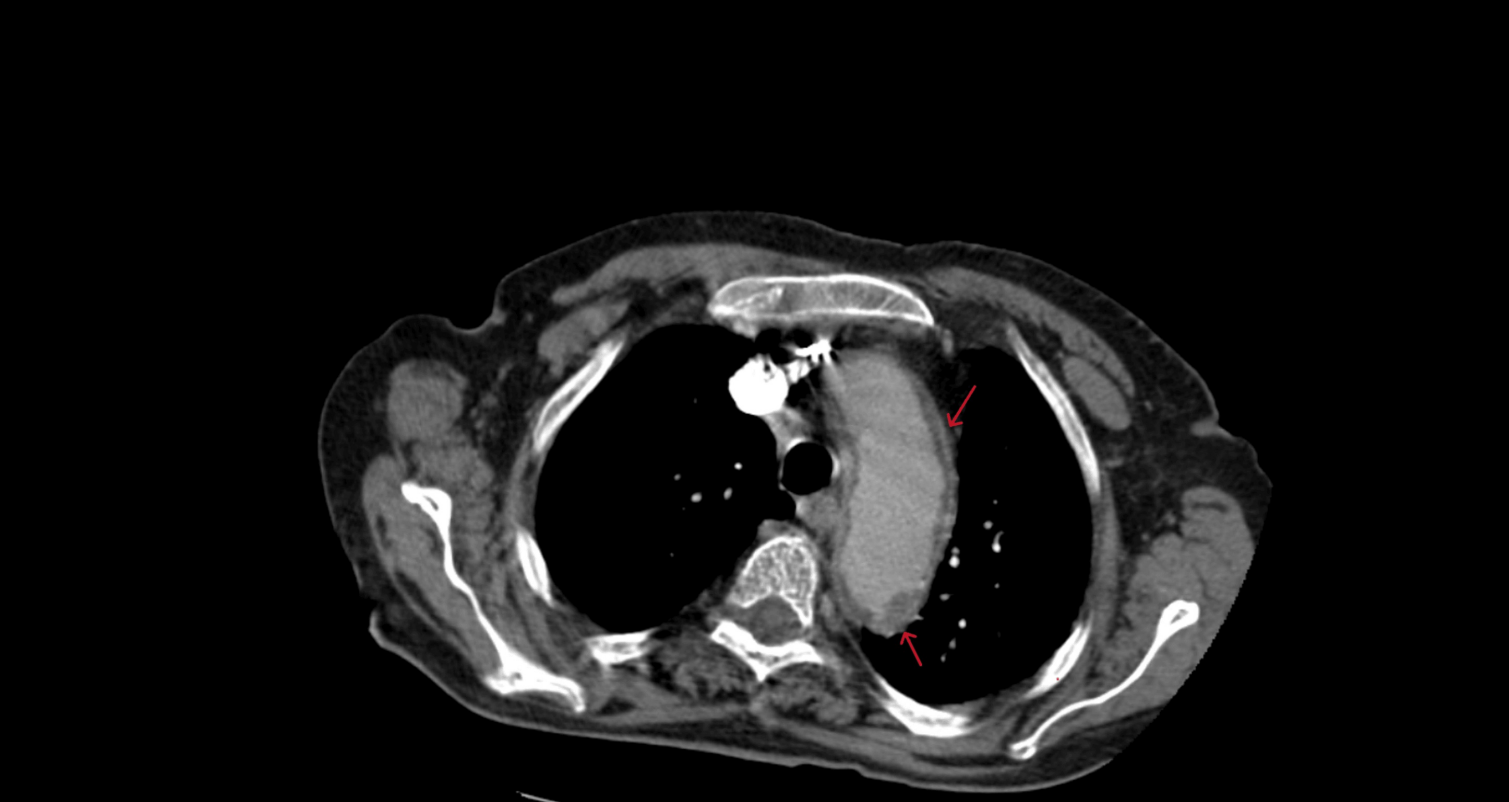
Computed tomography image of the chest (axial view) CT image showing circumferential infiltration of the aorta, known as “coated aorta” (red arrows).
